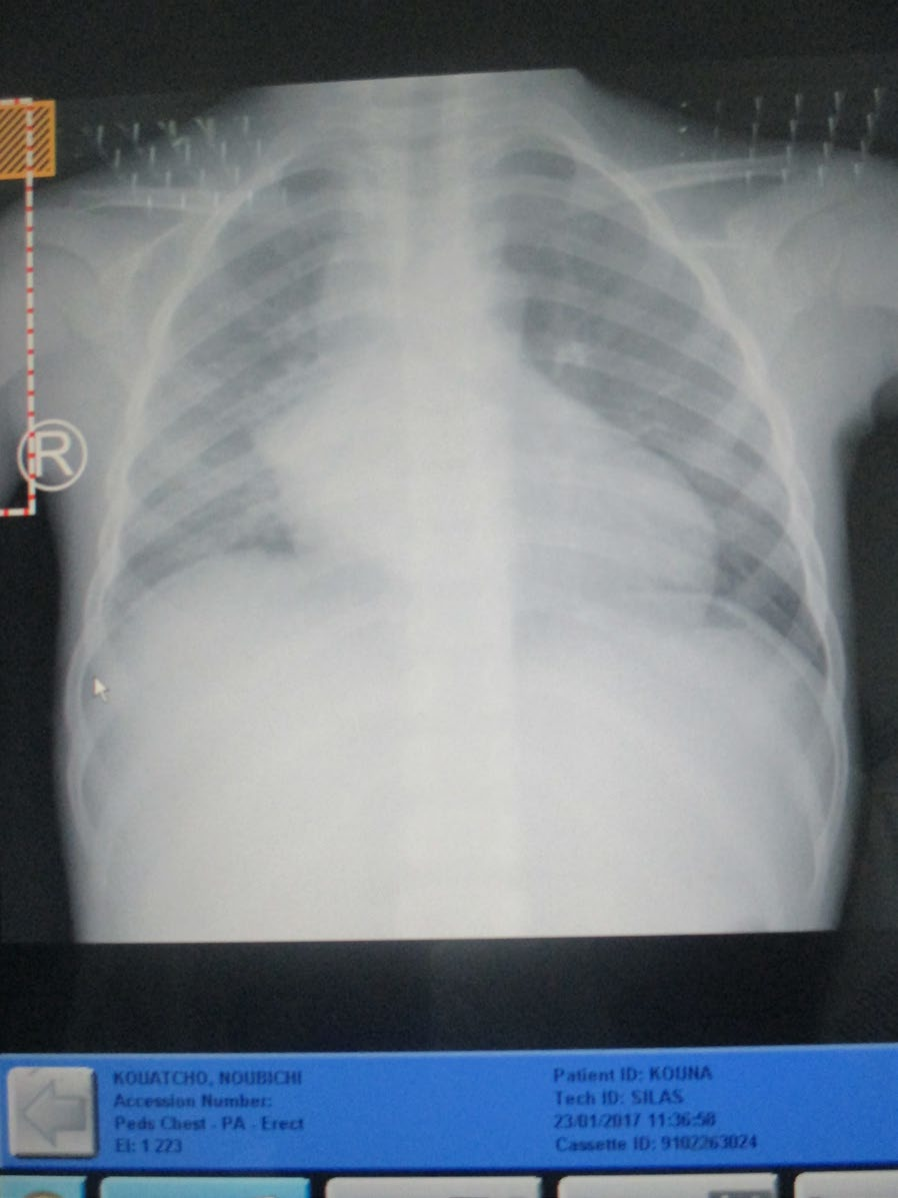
An antero-posterior view chest X-ray revealed a bilateral micro-nodular interstitial opacity associated with bilateral hilar adenopathy
